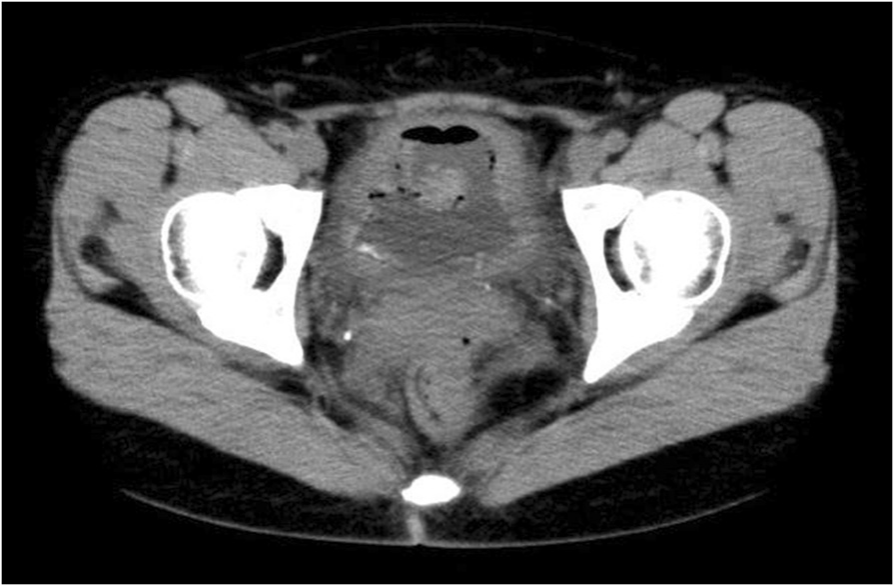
Computed tomography imaging demonstrating thickening of the whole bladder wall and spotty high-density lesions of the bladder surface, with clotting blood. A reduction in bladder capacity was suspected.
